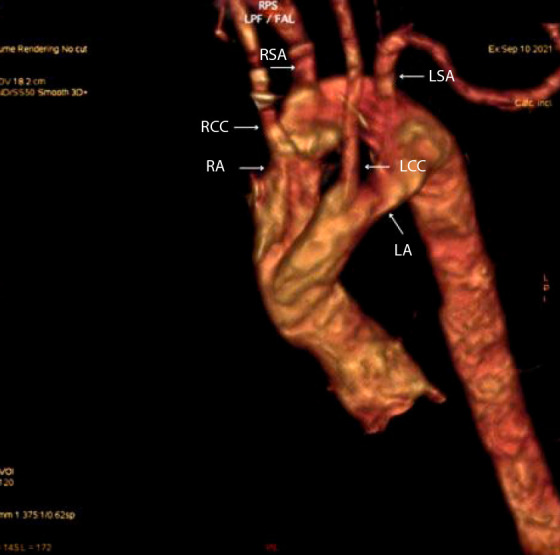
Contrast-enhanced chest computed tomography angiography with three-dimensional reconstruction, left anterolateral view. A complete double aortic arch forming a vascular ring is observed, with the right aortic arch (RA) being of larger caliber. The right (RCC) and left (LCC) common carotid arteries, as well as the right (RSA) and left (LSA) subclavian arteries, are identified. No brachiocephalic trunk is present. LA: left aortic arch
